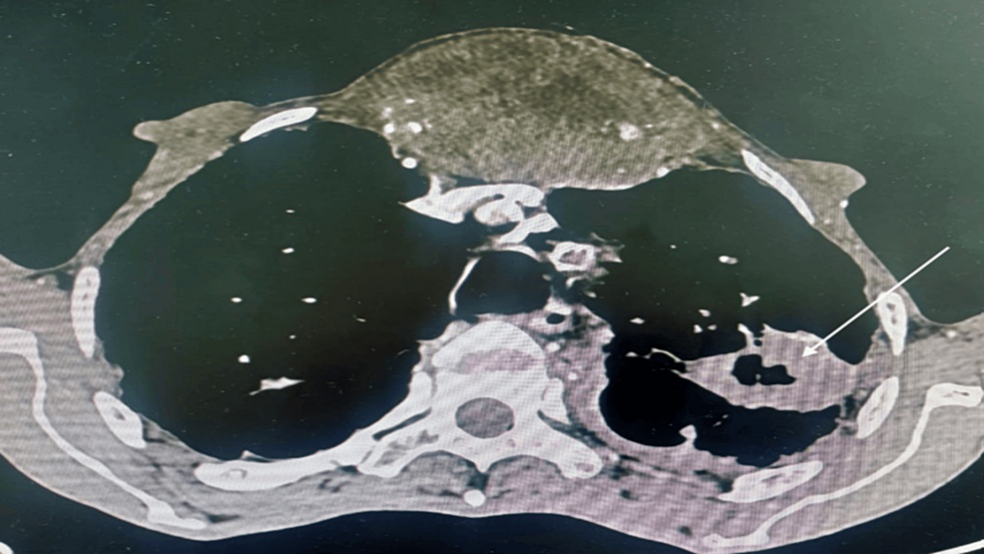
Chest CT scan showing an excavated lingular lung mass (white arrow)
